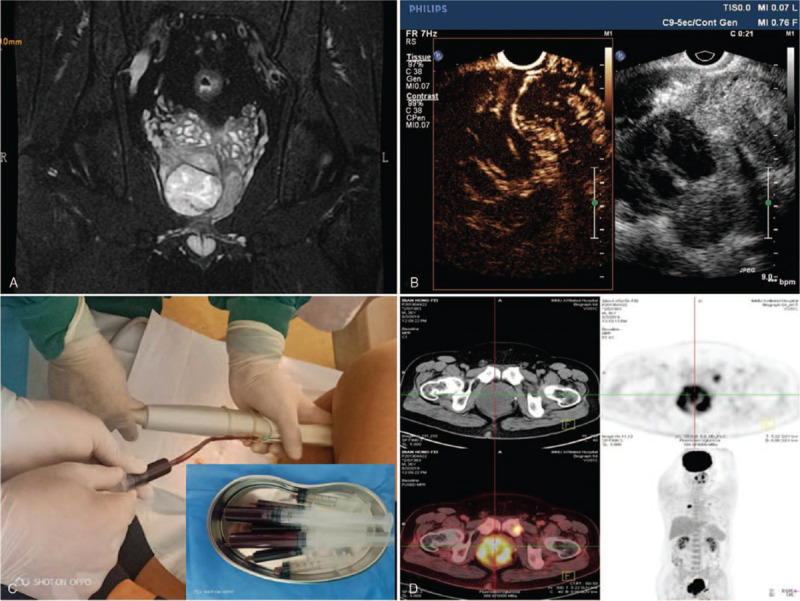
Imaging findings of Ewing's sarcoma. MRI revealed that the prostate was enlarged and the internal signal was uneven. A necrotic cystic area with long T1 and T2 signals was seen in the center (A). TR-CEUS showed that the peritumoral parenchyma enhanced rapidly and that the large blood vessels in the necrotic liquefying area enhanced rapidly and synchronously with the prostate parenchyma (B). According to the enhanced area shown by CEUS, a biopsy was performed, and 65 mL of dark red plasma was drawn out (C). PET/CT showed that the patient had bone metastases in the left iliac bone (D). MRI = magnetic resonance imaging, TR-CEUS = transrectal contrast-enhanced ultrasonography.
